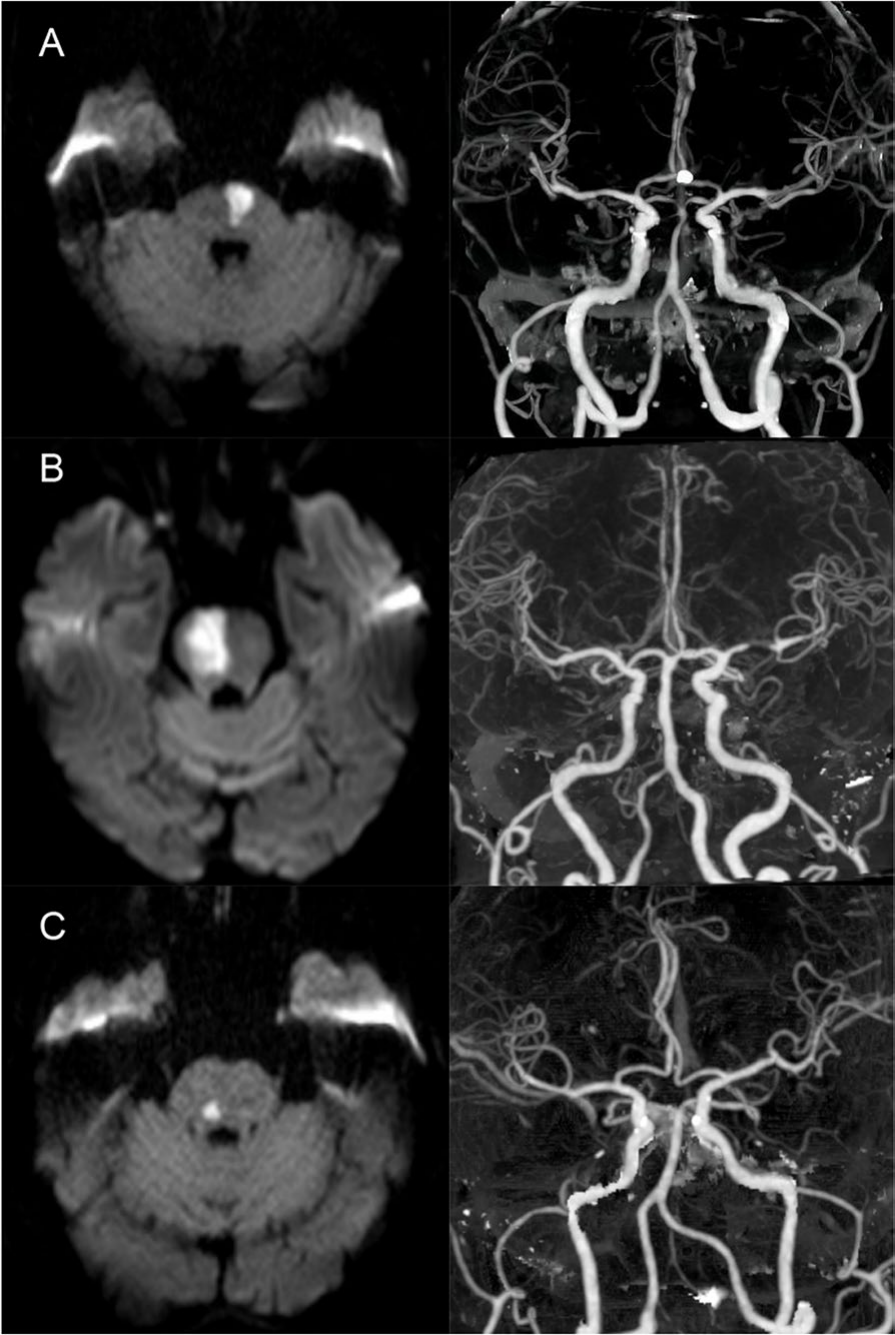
Examples of Isolated pontine infarctions. (A) PPI. DWI showed an infarction extending to the surface of the pons with CTA showing ≥50% stenosis in the basilar artery. (B) PPI. DWI showed an infarction reaching the pontine surface with irregularities of the basilar artery wall seen on CTA. (C) SDPI. DWI showed a lacunar infarction not reaching the basal surface of the pons without vertebrobasilar artery stenosis seen on CTA
